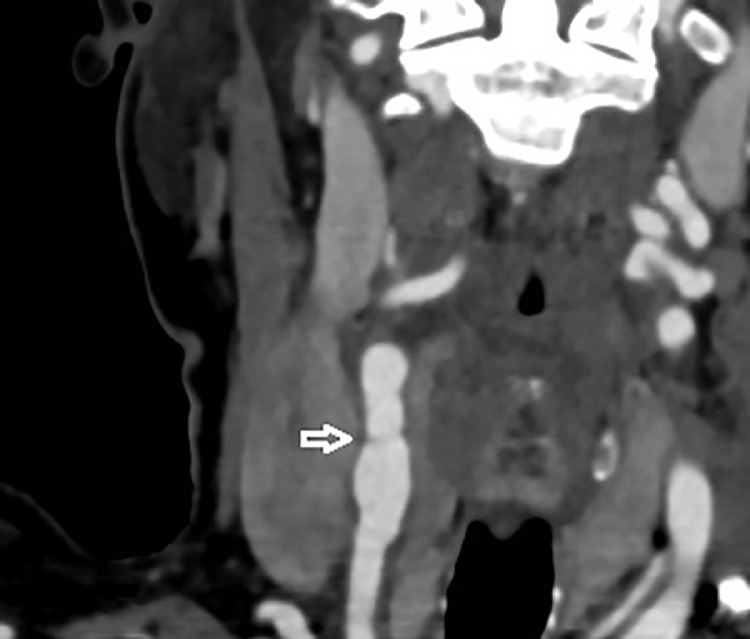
CTA showed a transverse low desnity strip ( arrow), causing luman stenosis with proximal post CCA aneurysmal enlargement on lower part of right.
